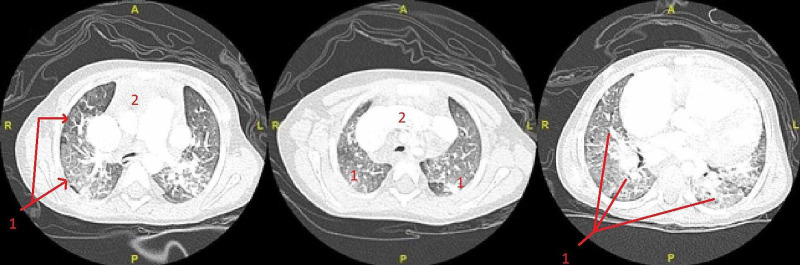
CT of the chest with IV contrast utilizing the cardiac great vessels protocol. 1: chest consolidation; 2: cardiomegaly
